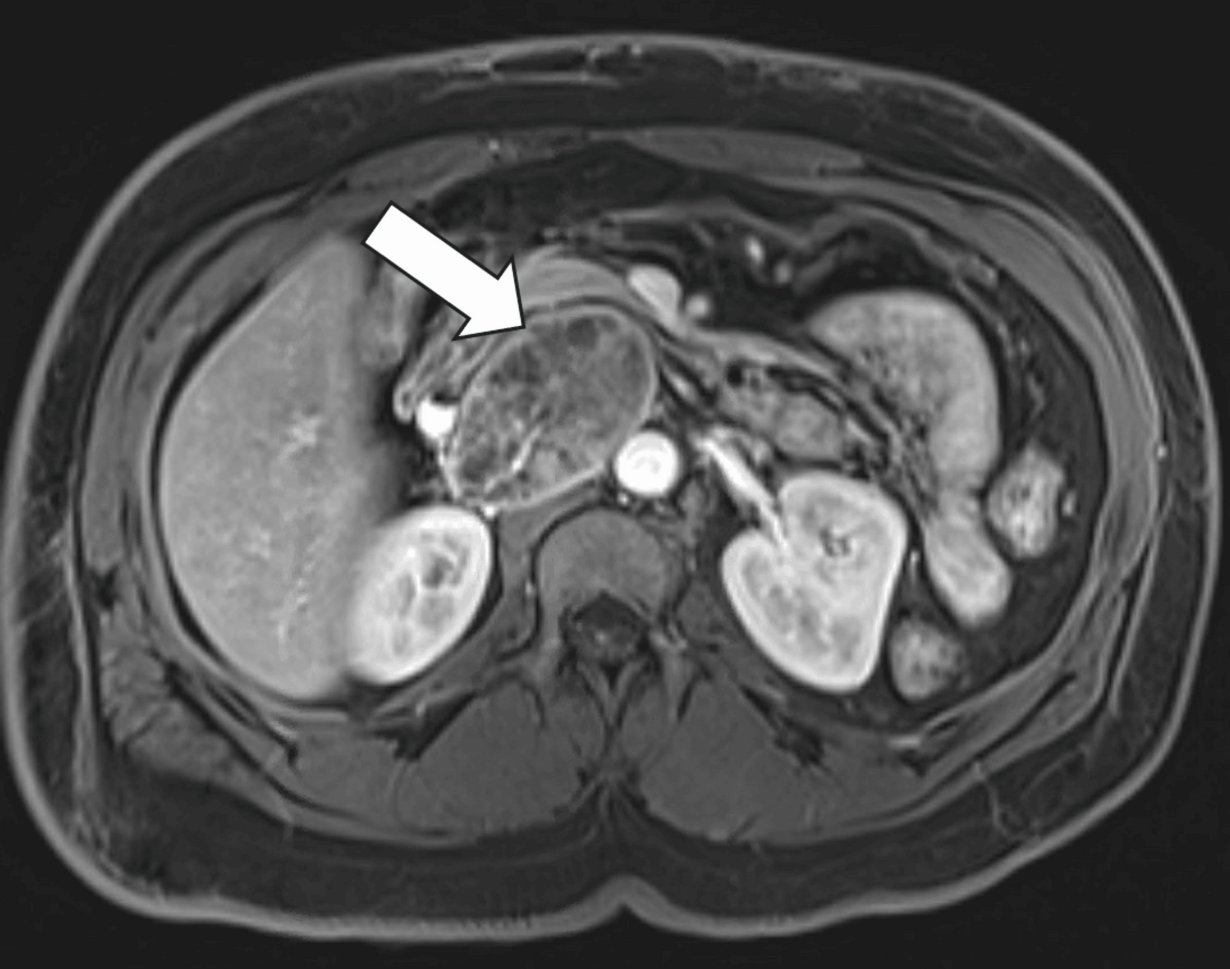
T2-HASTE (half-Fourier acquisition single-shot turbo spin-echo) MRI abdomen showing a 6.4 cm right retroperitoneum mass
